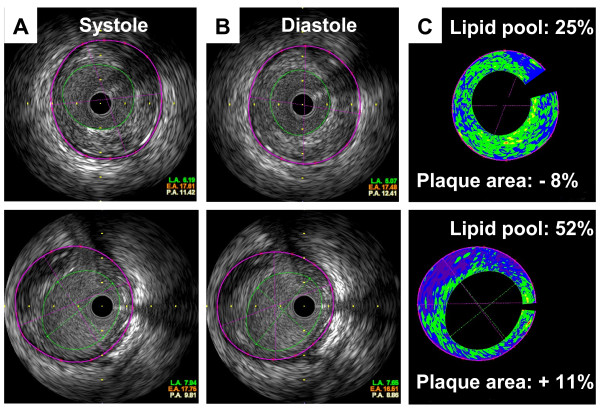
Intravascular ultrasound images of coronary arteries. (A) Gray scale images in systole. (B) Gray scale images in diastole at the same site as (A). (C) Integrated backscatter intravascular ultrasound images at the same site as (A) and (B). (Upper) Representative cross-sectional images that showed reduction of plaque area from diastole to systole. (Lower) Representative cross-sectional images that showed increment of plaque area from diastole to systole.
